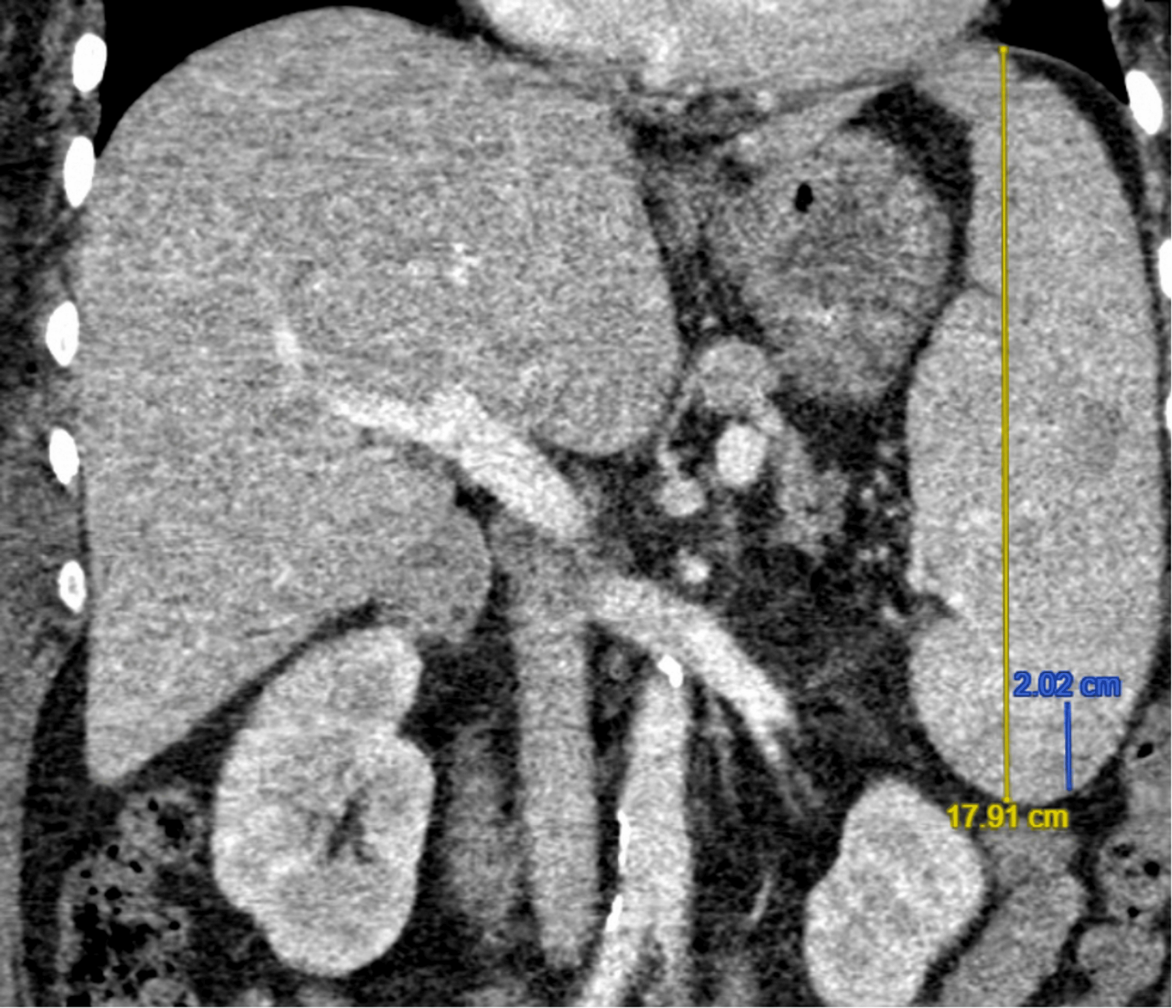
CT scan of the abdomen demonstrating splenomegaly, meeting one of the seven HLH-2024 criteria. HLH: hemophagocytic lymphohistiocytosis
